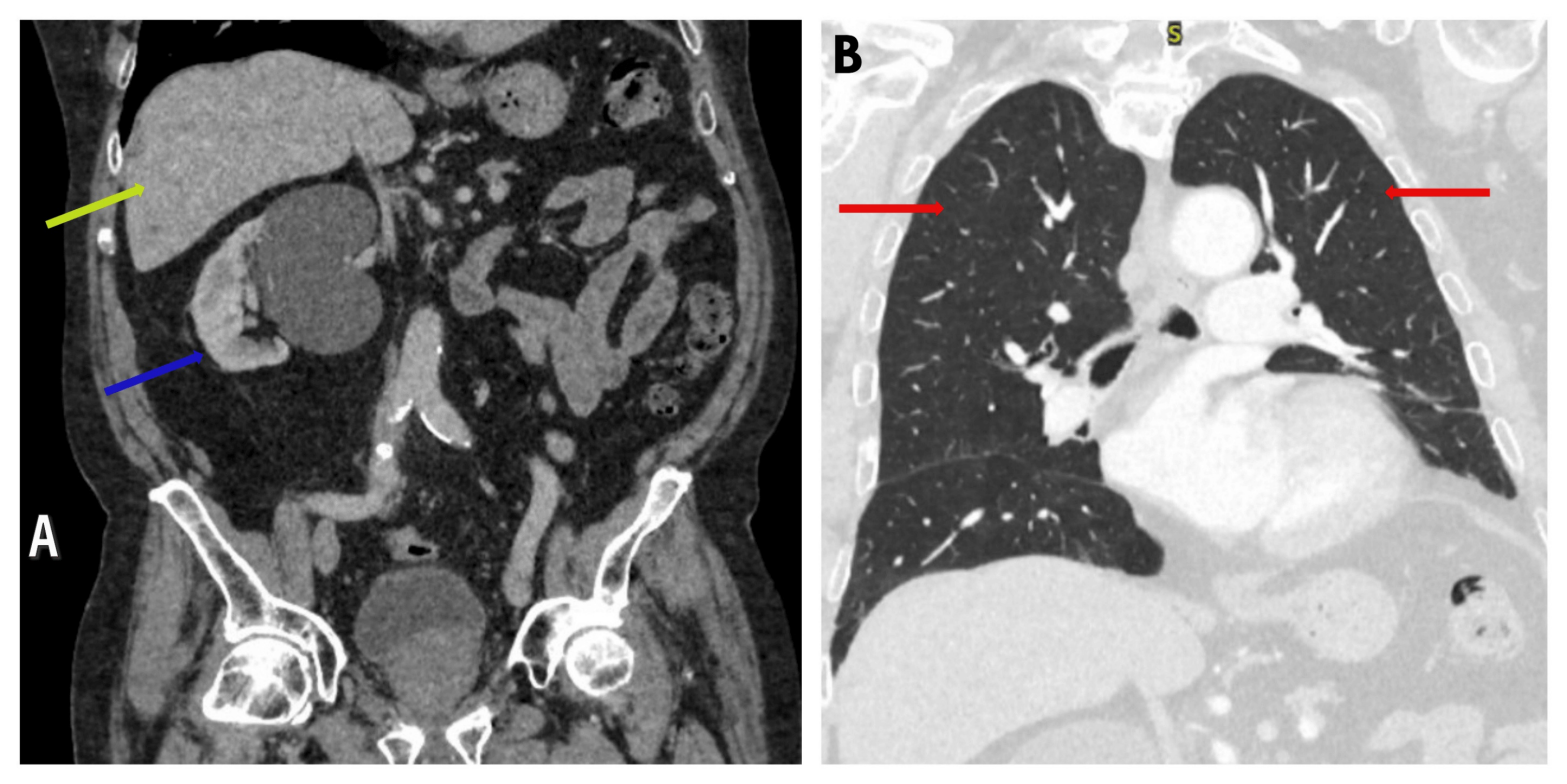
Six-month follow-up contrast-enhanced CT scan of the thorax, abdomen, and pelvis. (A) Abdomen and pelvis showing no metastasis. The yellow arrow indicates the liver, and the blue arrow indicates the right kidney with a simple cyst. (B) Chest scan showing no metastasis (red arrows point to the lungs).
